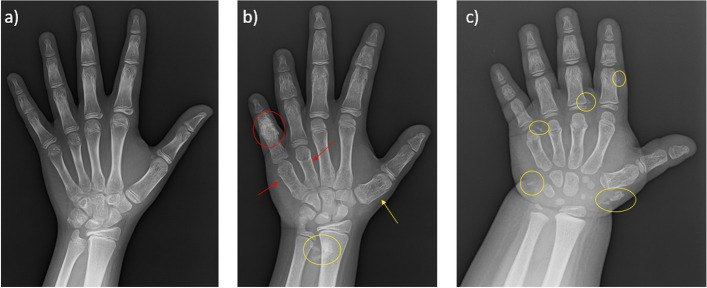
Bone age of reported patients. a Left hand and wrist x-ray of the patient II:1 without significant bone alterations. b Left hand and wrist x-ray of the patient II:2. Brachydactyly of the IV and V metacarpus (red darts). Osteopenia and swelling of the metacarpal of the first finger (yellow dart). Osteosclerosis of the proximal phalanx of the V ray with disappearance of the proximal interphalangeal space (red circle). Large soft tissue ossifications close to the distal radius and ulna epiphysis (yellow circle). c Left hand and wrist x-ray of the patient II:3. ossifications in the context of soft tissues of I, II and V finger (yellow circle)
